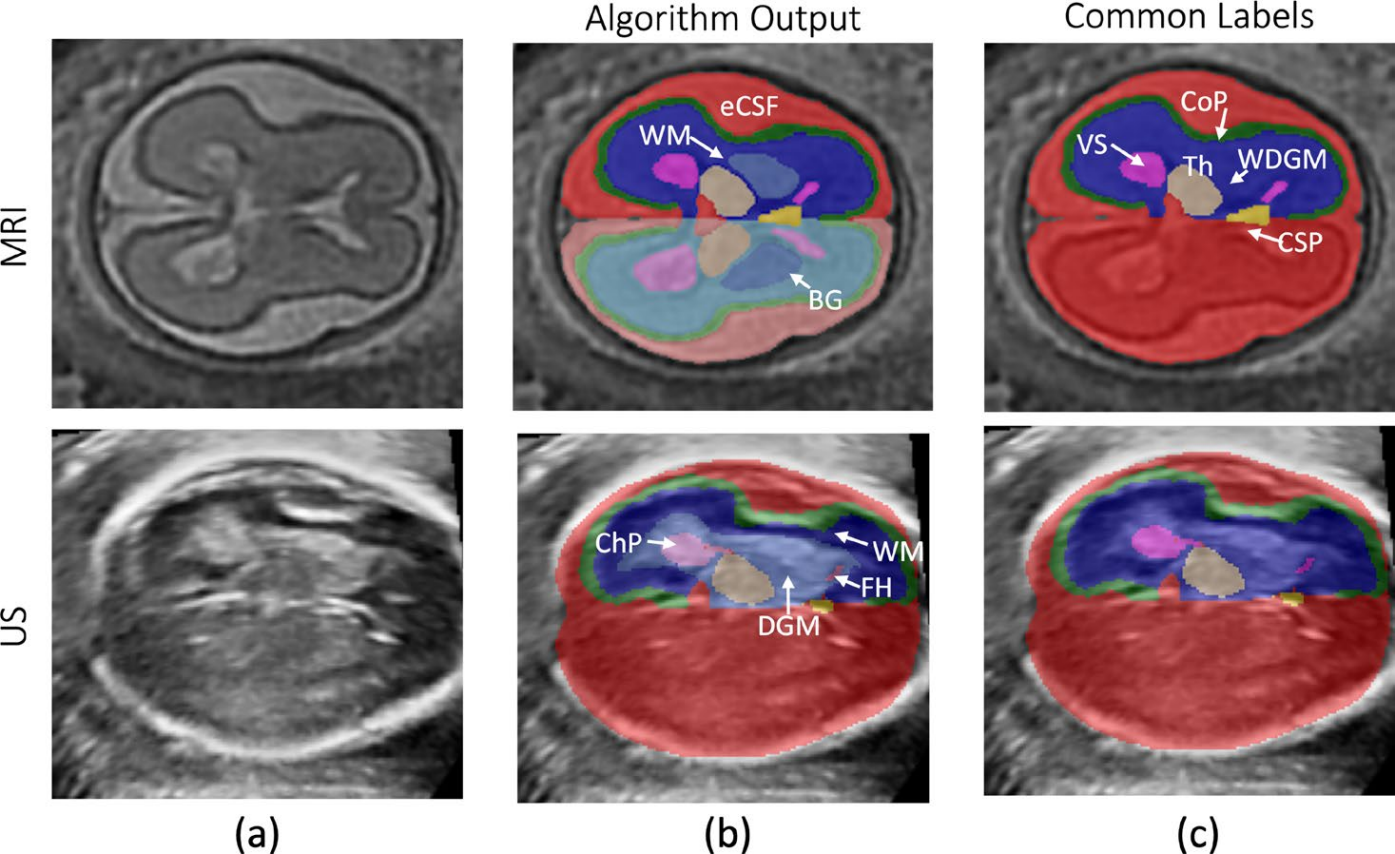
(a) Tissue labelling for a MRI and US scan pair at 25GW. (b) The output from the MRI and US deep‐learning algorithms, with the arrows pointing to structures that differ between the two outputs. (c) The common labels, generated by combining structures from the middle columns. Only the distal hemisphere (furthest from the US probe) was used, as large acoustic artefacts obstruct the anatomy in US on the proximal hemisphere. A full breakdown of combined labels to generate the common labels is shown in Table S1. The arrows are pointing at the following anatomical labels: White matter (WM), extracerebral cerebrospinal fluid (eCSF), basal ganglia (BG), choroid plexus (ChP), frontal horns (FH), deep grey matter (DGM), ventricular system (VS), thalamus (Th), white and deep grey matter (WDGM), cortical plate (CoP) and cavum septum pellucidum (CSP).
